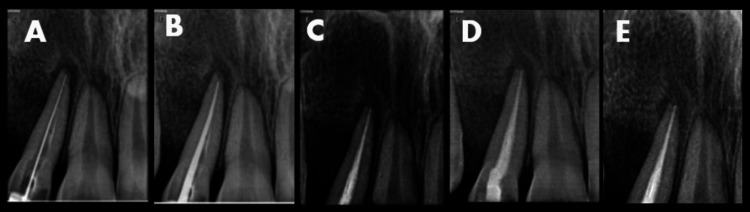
(A) Working length radiograph. (B) Master cone radiograph. (C) Obturation radiograph. (D) One-month review radiograph. (E) Six-month review radiograph.
